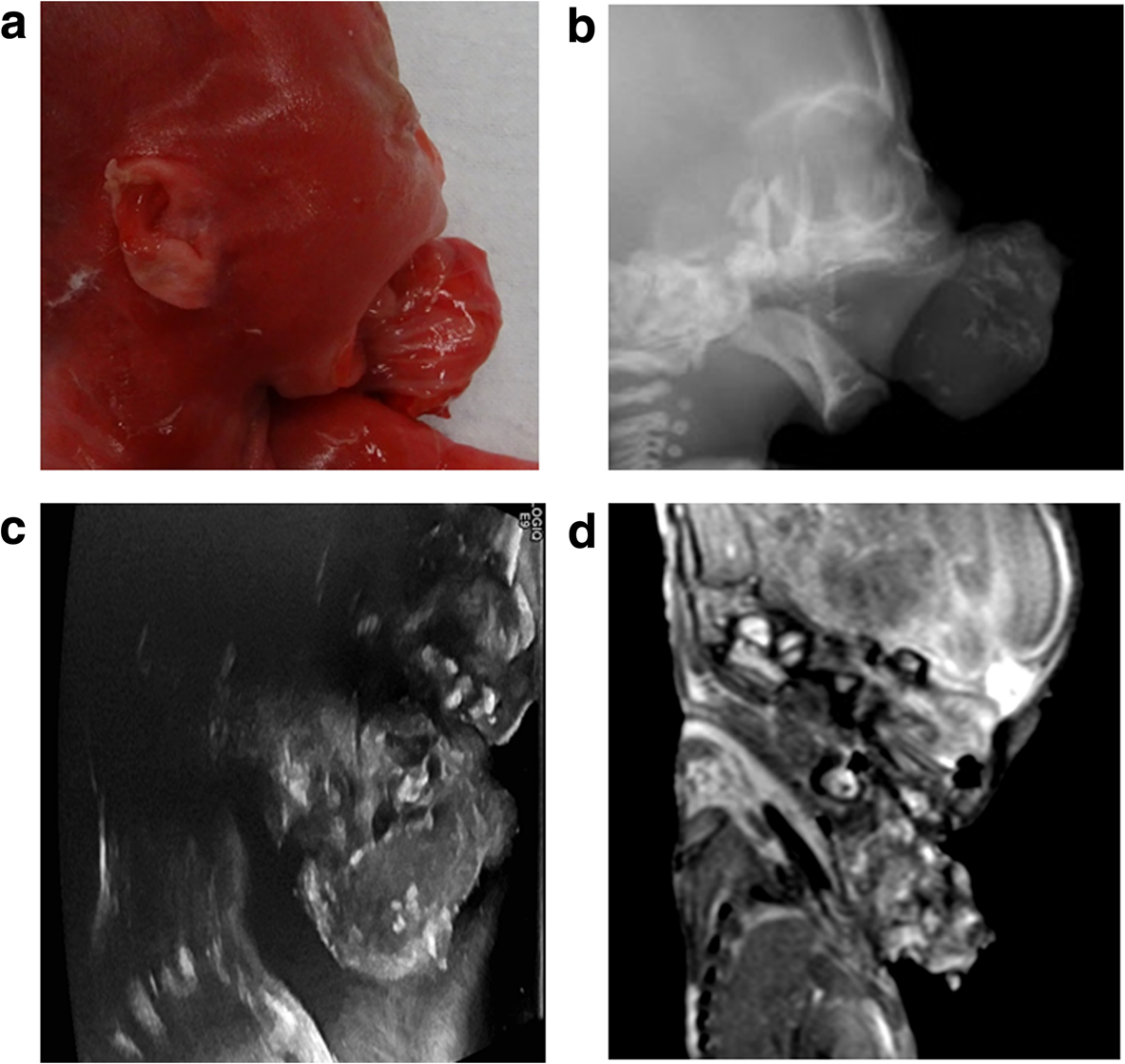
Epignathus arising from the hard palate in a foetus at 21 weeks gestation, after a termination of pregnancy for suspected anterior encephalocele. The photograph taken prior to autopsy of the head (a) demonstrates a large soft tissue mass arising from the oral cavity. The corresponding lateral radiograph of the head (b) demonstrates multiple calcific components within. A sagittal view during post-mortem ultrasound (c) and post-mortem MRI (d) obtained 7 days after delivery, both demonstrate the heterogenous internal nature of the oral mass, although it is much harder to identify the origin of the mass on the PMMR given the position, resolution and slumping of the foetus in the scanner
